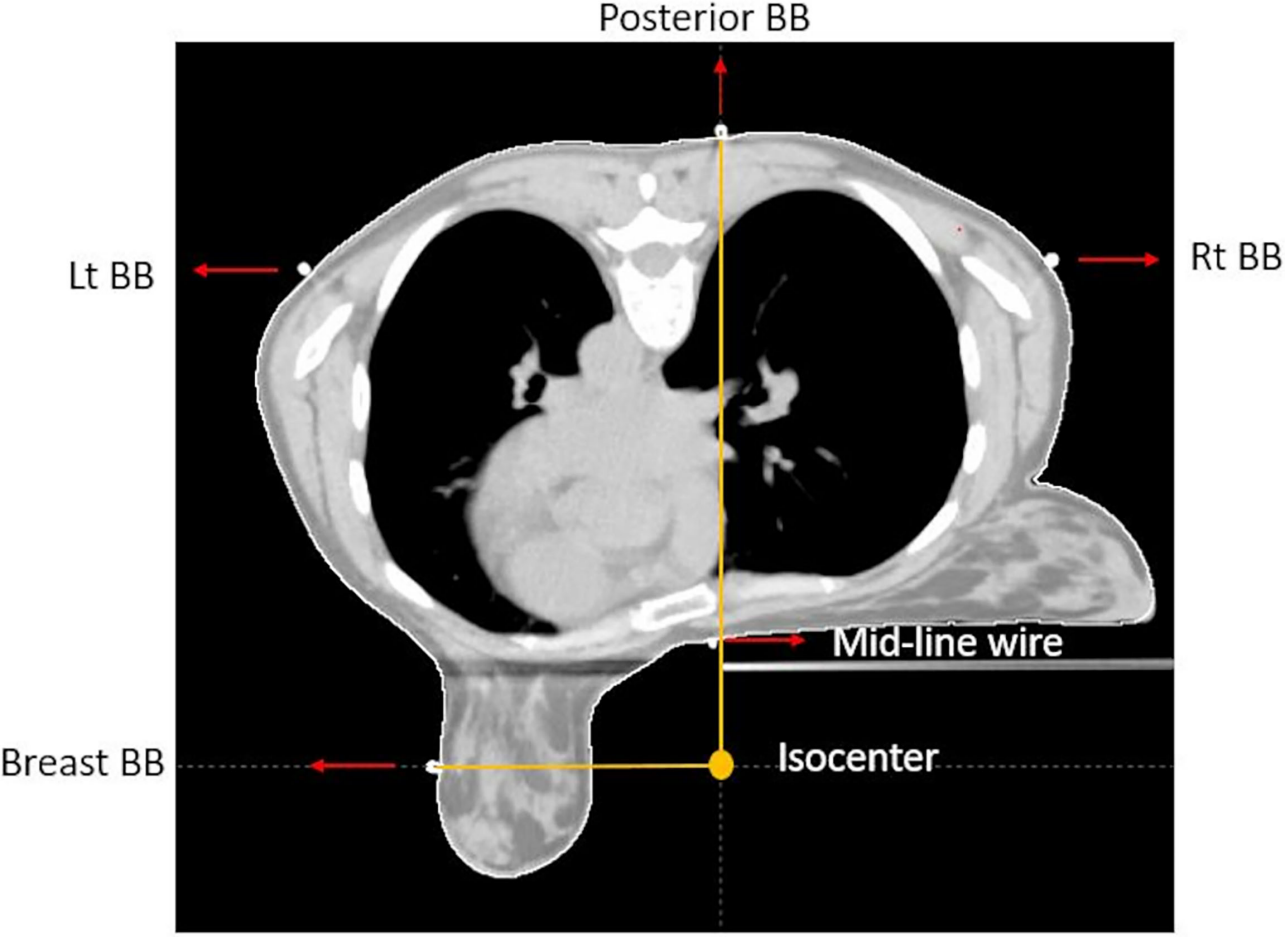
CT axial view of prone breast, showing isocenter location, triangulation point, lateral aspect of the breast marker, and midline marker.
